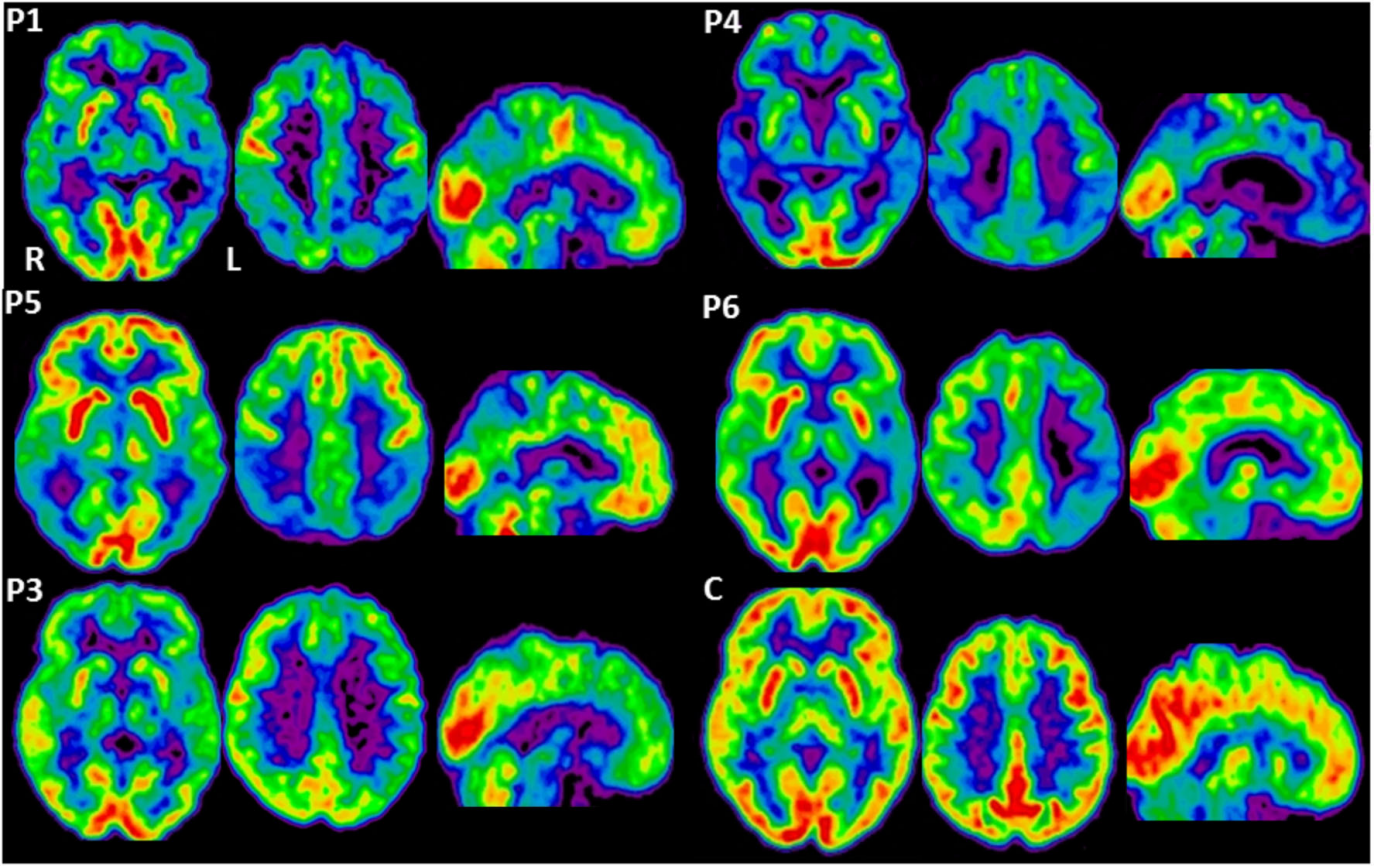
PET transaxial images at two axial and one sagittal section show ^18^FDG uptake in the patients (P1, P4, P5, P6, P3) and in a control subject (C). Compared with control, P1, P4, and P5 showed a relative hypometabolism involving mainly the posterior cortical regions including the PCC with some spread to the frontal cortex in P1, P4 and to the striatum (R < L) in P4. P6 and P3 show less marked asymmetric hypometabolism (L < R) involving also the striatum (L < R)
